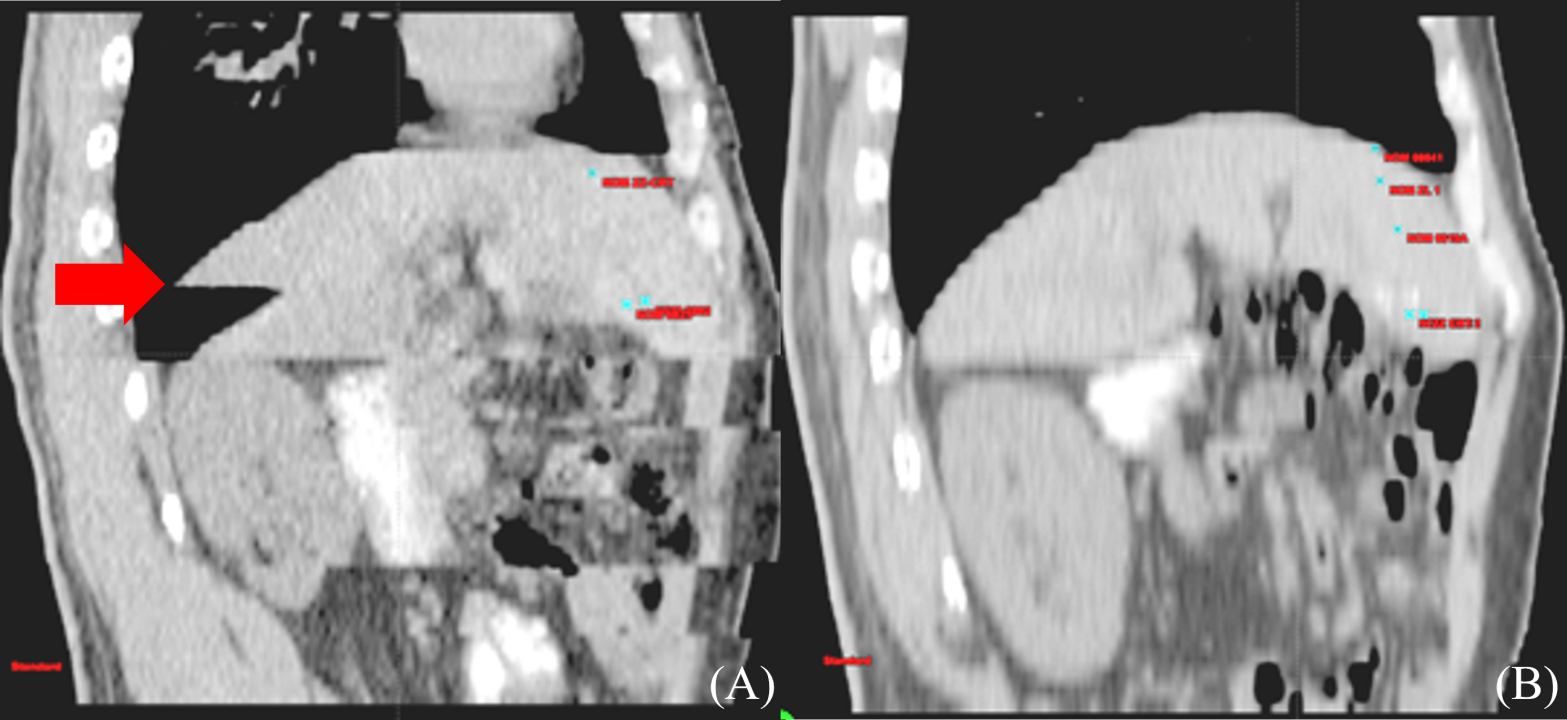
Differences of liver delineation imaging between free breathing and calm end-expiratory breath-hold CT scans. (A) CT sagittal imaging under free breathing (B) CT sagittal imaging at end-expiratory breath-hold phase.
